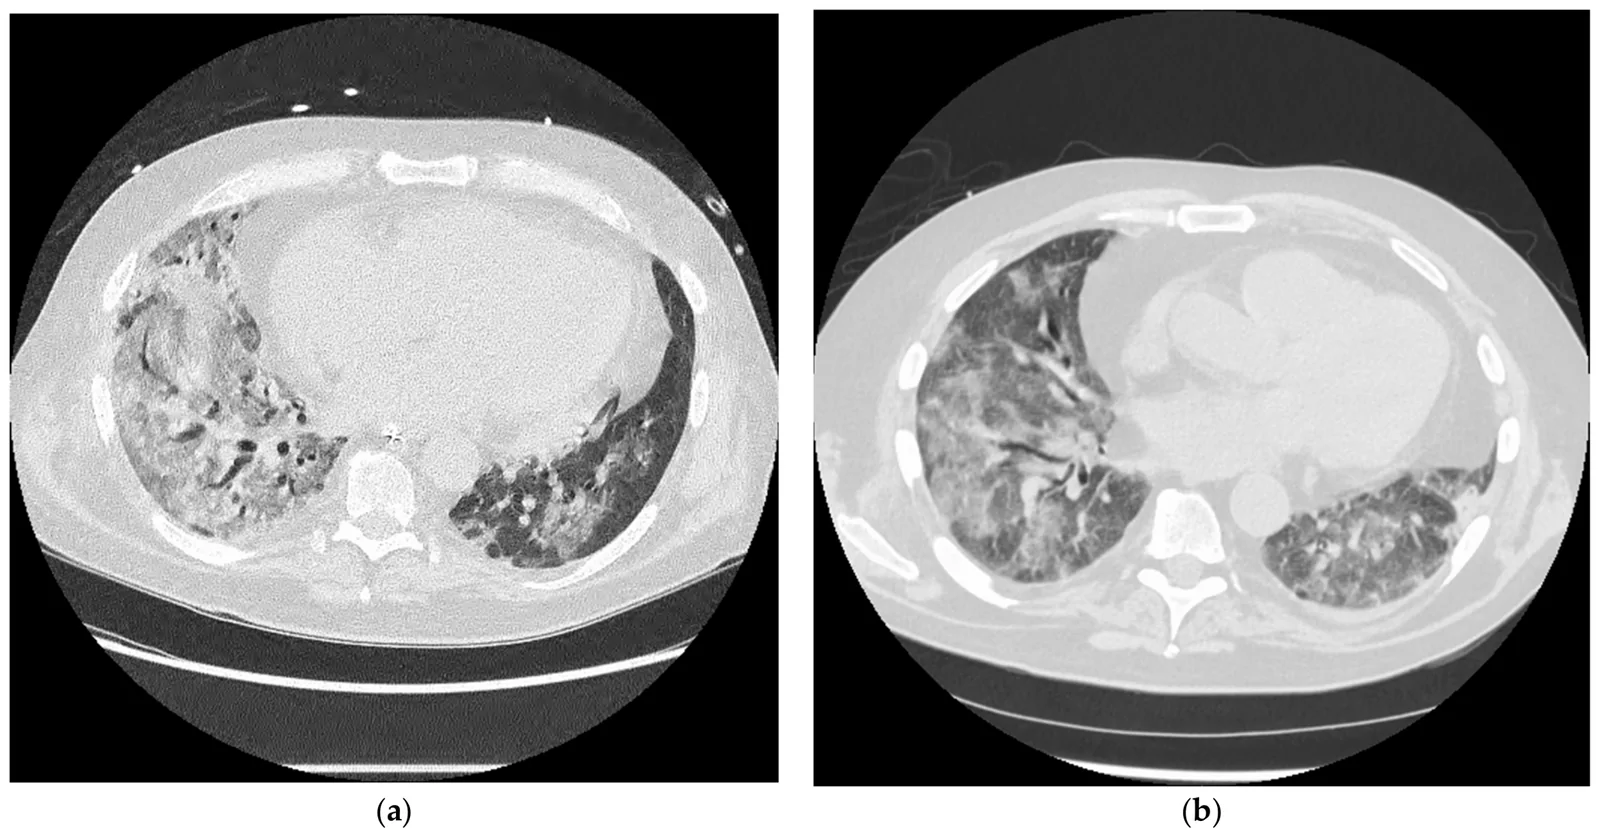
(a) Computed tomography of the chest without contrast demonstrating increased patchy consolidation in the right upper and middle lobes with adjacent ground-glass opacity, obtained during the second intensive care unit admission prior to anakinra initiation (authors’ original work). (b) Computed tomography of the chest without contrast demonstrating interval decrease in patchy ground-glass opacities throughout both lungs with interval increase in diffuse bronchiectasis, obtained following anakinra therapy. Compare with Figure 1a (authors’ original work).
